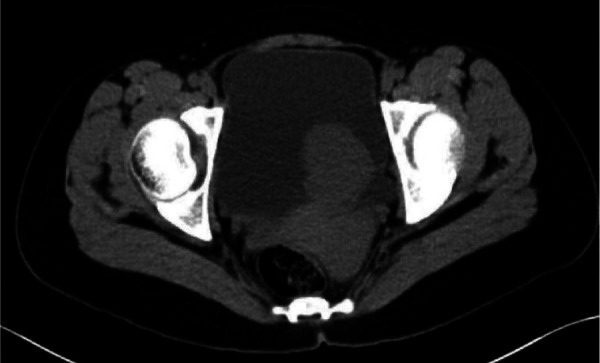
The unenhanced CT scan of the patient whose PUB tumor was located in left posterior wall.
